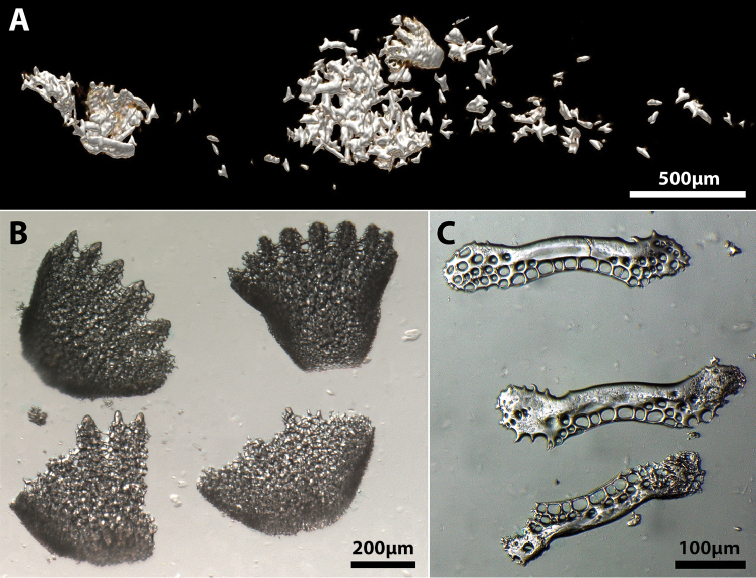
Gut content of *Flexorincus.*A Hard gut content of AIM MA655316, paratype, 23.0 mm SL, as revealed by CT scanning. Isolated elements from the gut of AIM MA655142, paratype, 20.0 mm SLB Stereomic ossicles tentatively identified as elements from the terminal disc of an echinoid C Rod-like elements tentatively identified as ossicles from the body of a holothuroid.
